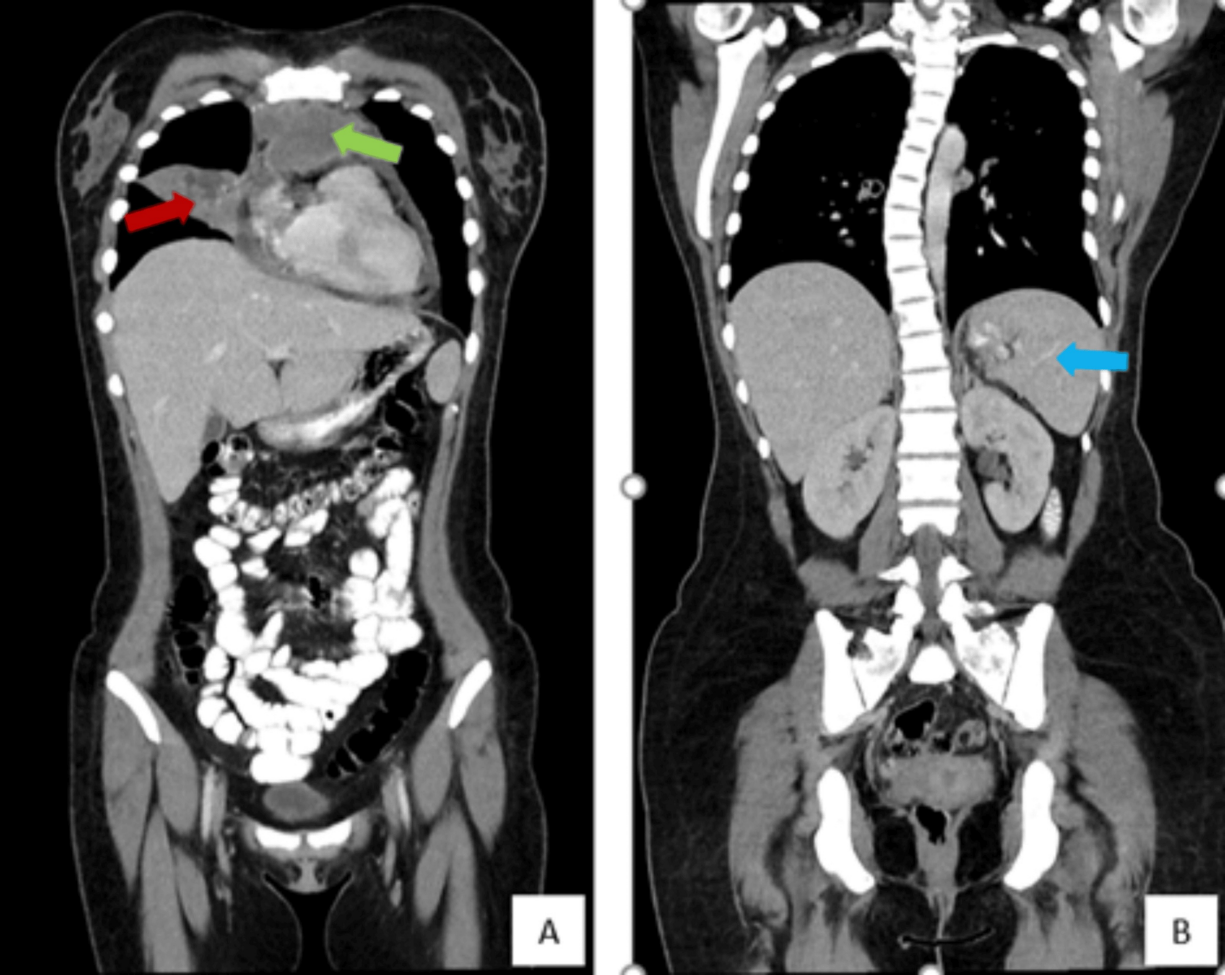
Thoraco-abdominopelvic computed tomography - coronal plane. (A) Lymphadenopathic involvement of the mediastinum, particularly in the thymic region, with a central necrotic component extending over an 8.8 cm area (green arrow); tumoral involvement of the pulmonary parenchyma in the middle lobe with a large area of consolidation and a necrotic center measuring 9 cm at its longest axis (red arrow); (B) Splenomegaly with a maximum axis of 13.7 cm (blue arrow).
